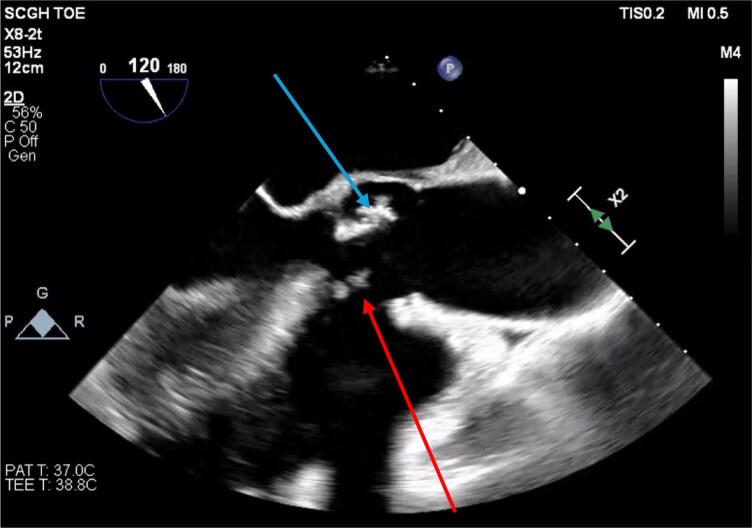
Intraoperative transoesophageal echocardiography image in the mid-oesophageal long-axis view (120°) demonstrating the aortic root and ventricular septal defect. The blue arrow indicates vegetation on the aortic valve, whilst the red arrow identifies the ventricular septal defect.
